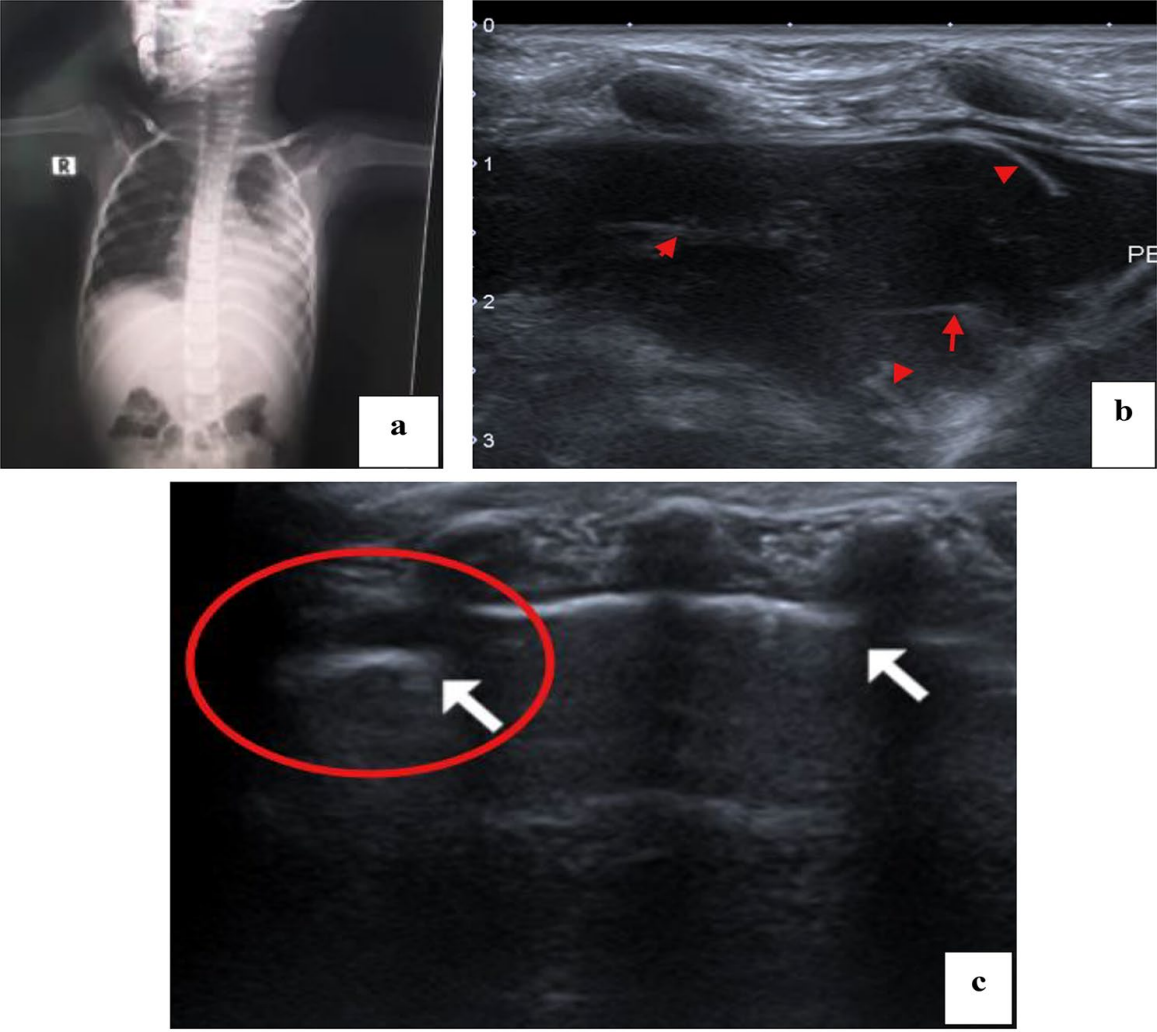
A 5-year-old female child with complicated community-acquired pneumonia. (a) The initial plain x-ray done on admission showed an obliterated costophrenic angle with the opacification of the lower part of the left hemithorax. (b) Lung ultrasound examination done with the probe placed at the left costophrenic angle showing moderate pleural collection/effusion with internal echogenic bands (the arrows represents the bands). (c) Lung ultrasound examination with the probe placed at zone 3 of the left lung, showing transition point on the lung surface where the lower arrow represents normal lung sliding, compared with absent lung sliding, the arrow within the oblong, lung point sign, denoting presence of pneumothorax
